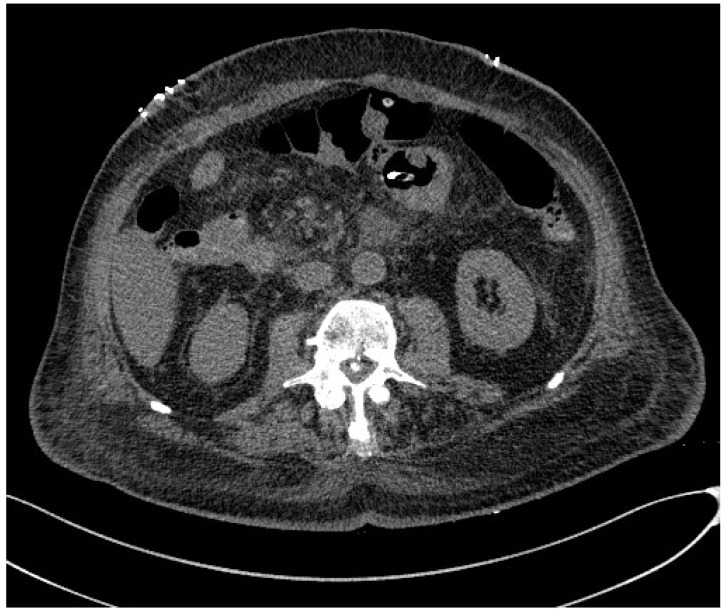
Non-contrast-enhanced CT scan of a sarcopenic patient with pancreatic carcinoma.
